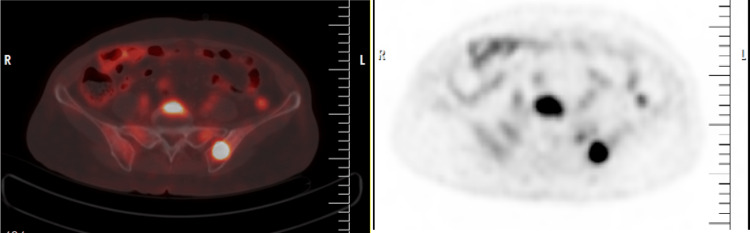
PET/CT PET/CT showing FDG-avid lesion with osseous involvement in a patient with diffuse large B cell lymphoma PET/CT, positron emission tomography/computed tomography; FDG, fluorodeoxyglucose
